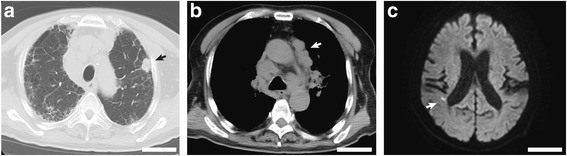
Radiologic images of the tumor and brain. a–b Axial computed tomography images show a 21-mm, solid, pulmonary nodule in the left upper lobe (a), arrow) and swollen mediastinal lymph nodes (b), arrow). c The axial diffusion-weighted magnetic resonance image shows a tiny high-intensity area (arrow) near the right-side ventricle in the cerebrum. Bars indicate 5 cm
